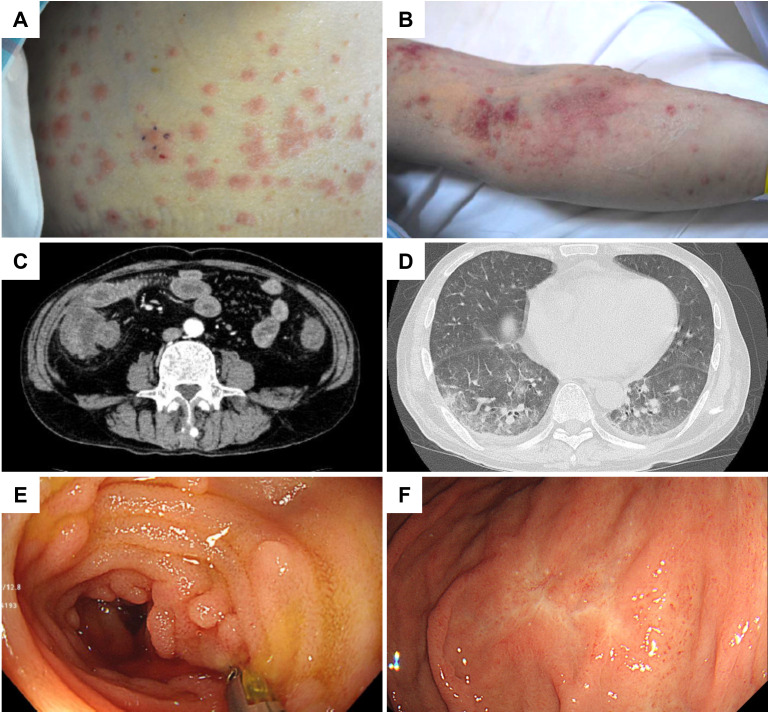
Clinical manifestations at referral. (A) Erythematous eruptions on the trunk. (B) Erythematous eruptions on the upper extremity. (C) Contrast-enhanced abdominal computed tomography showing diffuse wall thickening of the entire colon and distal small intestine. (D) Chest computed tomography showing bilateral ground-glass opacities. (E) Colonoscopy showing mucosal edema without ulceration in the terminal ileum. (F) Upper gastrointestinal endoscopy showing atrophic gastritis with multiple ulcer scars.
